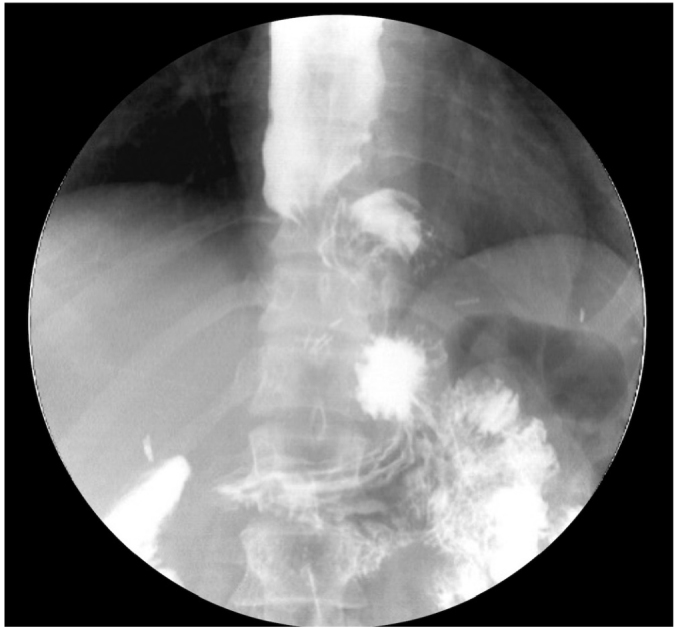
This contrast radiography shows a significant valve migration. Endoscopic procedures would not correct the valve migration, and ergo, were not considered. The patient was treated with a revisional laparoscopic surgery.
